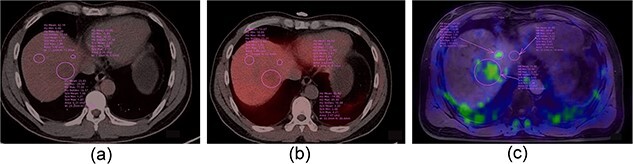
(a) [^18^F]-FDG PET/CT scan showing no obvious uptake. (b) [^11^C]-acetate PET/CT scan indicating slight uptake only for the largest lesion located in lobe VII (SUV_max_ = 4.21, TBR = 1.45), whereas other smaller ones show no uptake. (c) ^68^Ga-FAPI PET/CT scan not only shows strong, high uptake for the largest lesion (SUV_max_ = 1.94 TBR = 3.08), but also the smaller intrahepatic lesions (SUV_max_ = 1.47, TBR = 2.33). SUV_max_, maximum standardized uptake value; TBR, tumor-to-background ratio.
